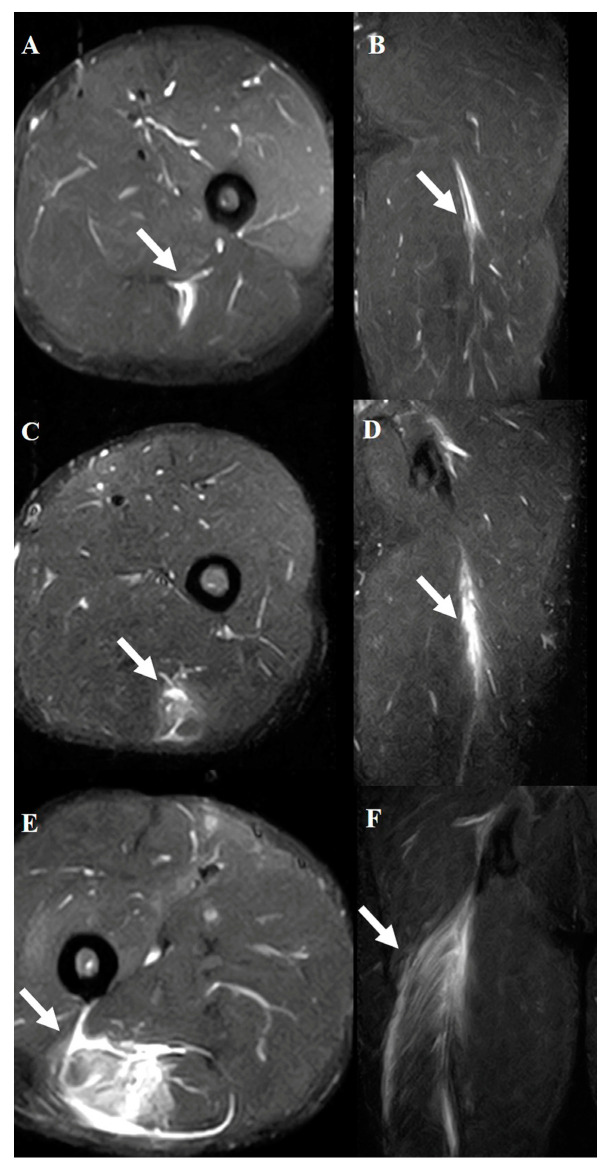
Typical MRI findings in acute hamstring strains. All views show a musculotendinous junction injury of the biceps femoris muscle. Axial (A) and coronal (B) views of grade 1. Axial (C) and coronal (D) views of grade 2. Axial (E) and coronal (F) views of grade 3. The white arrows indicate injured areas.
